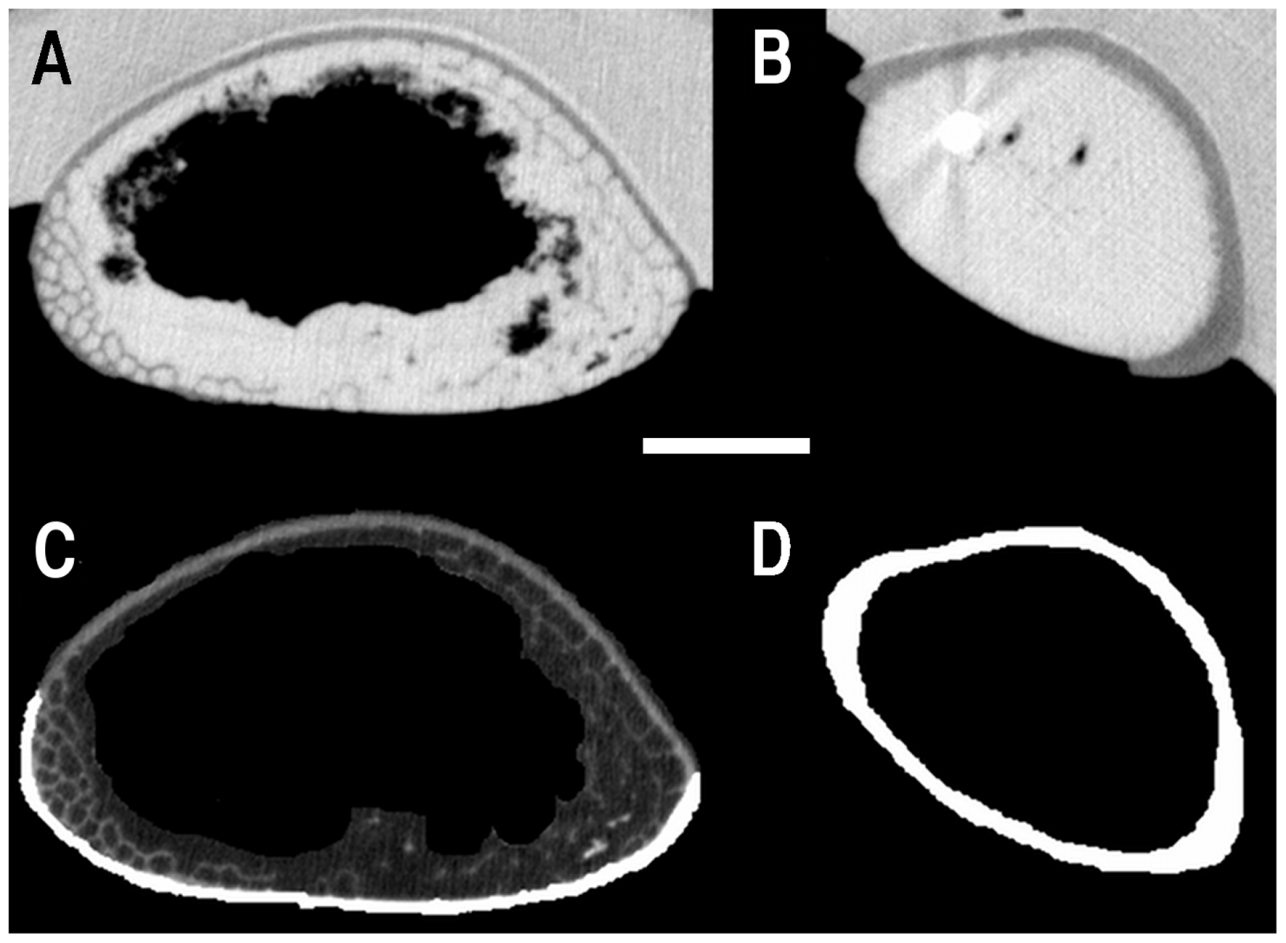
CT scan images from two different regions of pterosaur first wing phalanx. A and B show the unmodified CT scans from A) the distal end of UP WP1 and B) the mid-shaft of UP WP1, while C and D show the modified and corrected images used in the calculation. Air space proportion (ASP) is calculated by determining the cross-sectional area of the internal, air filled cavity (the black centre of D) and dividing that by the total cross-sectional area, including the white cortical tissue and the black cavity. In areas with trabeculae, like C, the calculation of the air space includes the air found in individual trabeculae around the edges. Scale = 10 mm.
